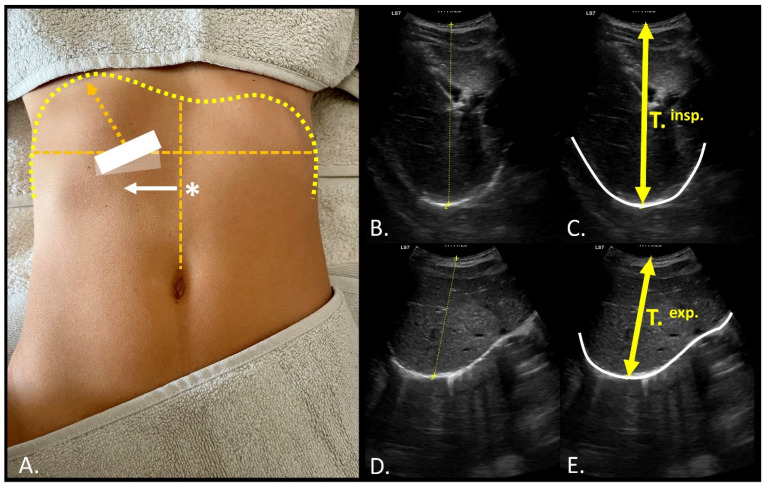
Assessment of diaphragmatic excursion using the transhepatic maneuver; (A) Using the umbilical line (*) as a reference, the probe is moved laterally to project directly onto the right diaphragmatic dome at the level of the 9th–10th ribs (white arrow). The probe is positioned at a tilt angle of 30–40° on the sagittal plane, with efforts to maintain the ultrasound beam projection as vertical as possible during respiratory phases. Pivot movements of the probe are adjusted to optimize visualization during the expiratory (B,C) and inspiratory (D,E) phases, while holding the probe firmly with both hands to minimize displacement. Abbreviations: T.^insp.^, inspiratory time during basal breathing; T.^exp.^, expiratory time during basal breathing.
